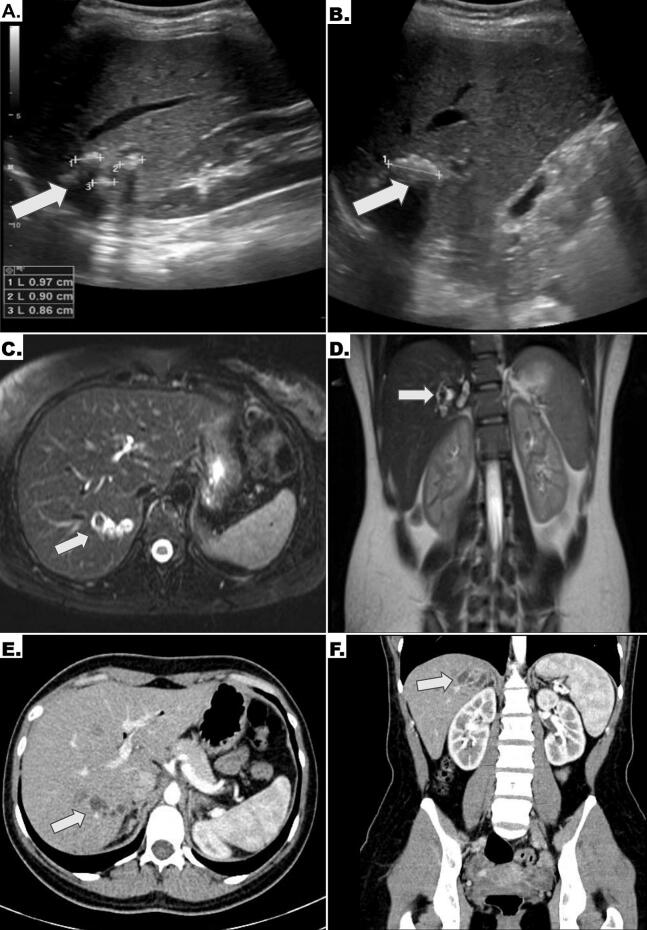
Imaging studies: Abdominal ultrasound: (A) Multiple hyperechoic foci with posterior acoustic shadowing, consistent with intrahepatic lithiasis. (B) A 3 cm hyperechoic lesion with similar characteristics located within the hepatic parenchyma. Gadolinium-enhanced magnetic resonance imaging (MRI): (C) Axial T2-weighted sequence showing hyperintense lesions associated with mild intrahepatic bile duct ectasia. (D) Corresponding coronal view of the same findings. Contrast-enhanced computed tomography (CT), arterial phase: (E) Axial and (F) coronal images showing ductal ectasia in hepatic segment VII, with no evidence of vascular malformations.
